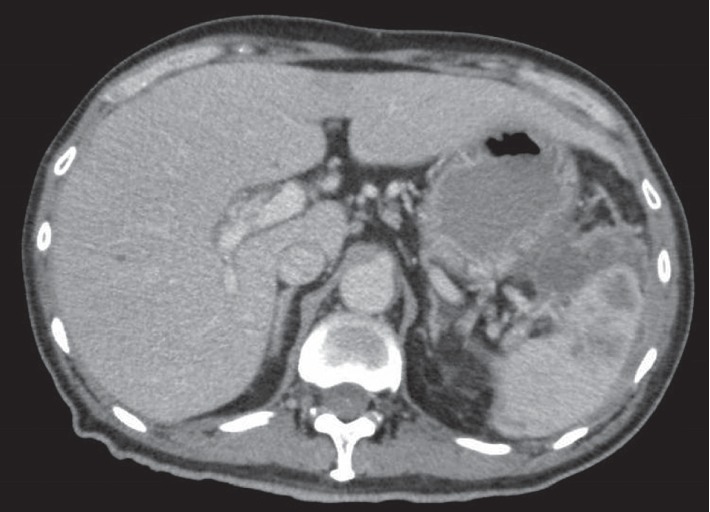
Computed tomography image of splenic abscess.
